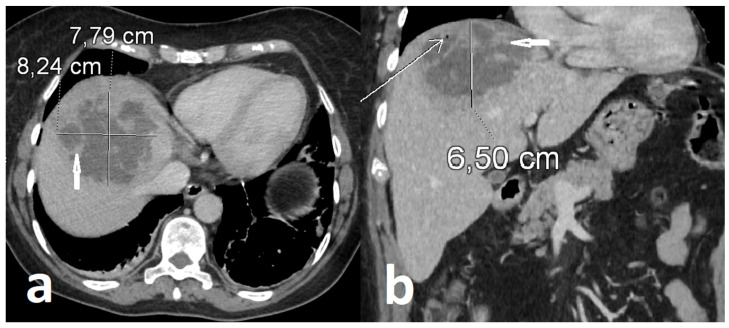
Hepatic abscess in initial contrast-enhanced CT scan measuring approx. 8.2 × 7.8 × 6.5 cm: axial (a) and coronal (b) plane views. Abscess located in the subdiaphragmatic region, reaching the surface of the liver with focal abutment of the diaphragm, contains irregular internal septa (short arrows) and intralesional gas (long arrow).
